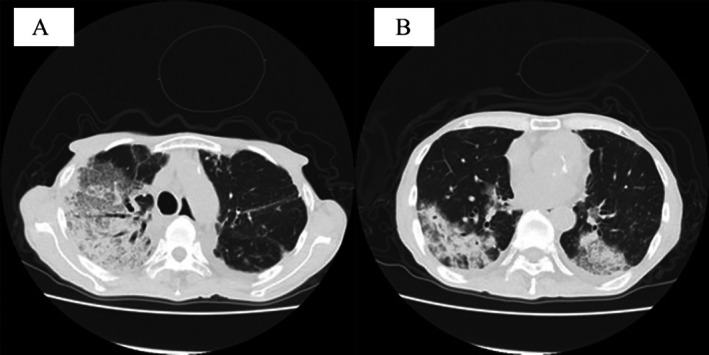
Computed tomography demonstrates consolidation involving the right upper to lower lobes (A), with additional consolidation noted in the left lower lobe (B). (A) CT image demonstrating extensive consolidation extending from the right upper lobe to the right lower lobe. (B) Additional consolidation in the left lower lobe indicating multilobar involvement in both lungs.
